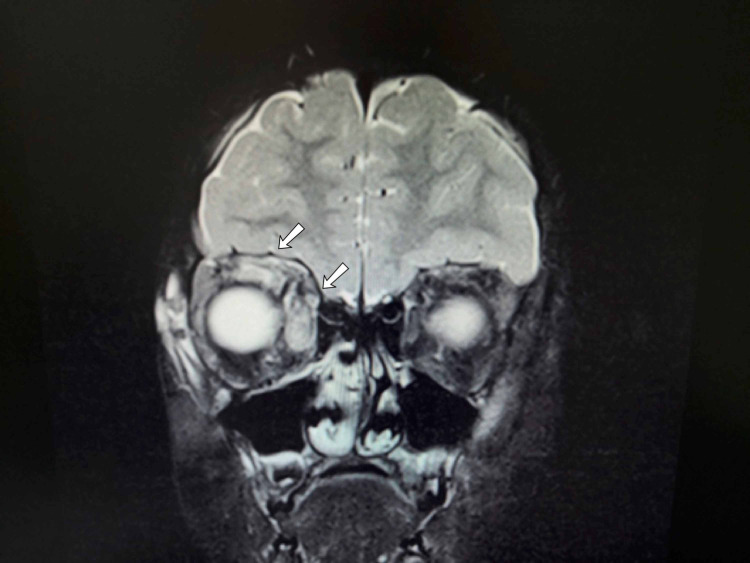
Coronal MRI orbits with gadolinium showing enhancing, thickened right superior and medial rectus muscles with tendon involvement. MRI, magnetic resonance imaging
